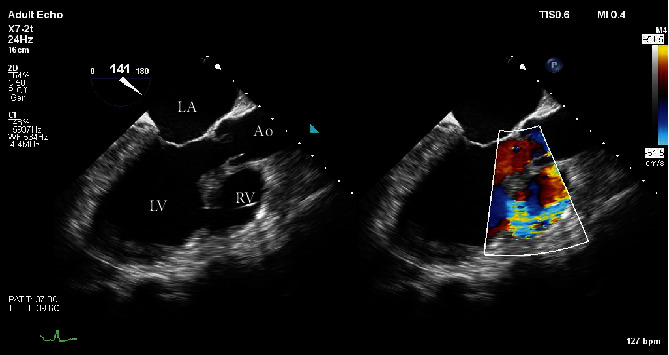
Transesophageal echocardiogram, midesophageal 3-chamber view taken whilst on Impella support, showing large VSD with left-to-right shunting on colour Doppler.
